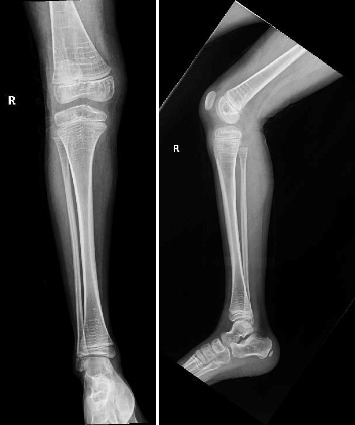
Radiograph of the legs at 6 years of age.
